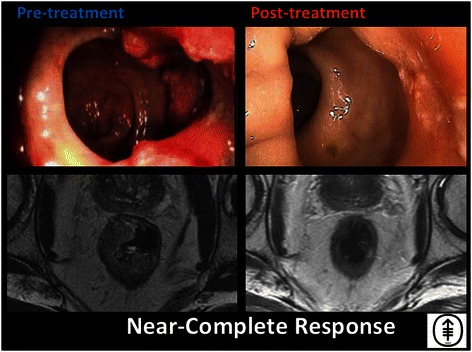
Near-complete response. Endoscopic and T2-weighted MRI images both pre- and post-treatment are shown for a patient who has achieved a near complete response. This is a 74-year-old man who underwent 8 cycles of induction FOLFOX followed by CRT, and achieved a near-cCR. A biopsy obtained in surveillance was determined to contain residual cancer; therefore, the patient was referred for TME
